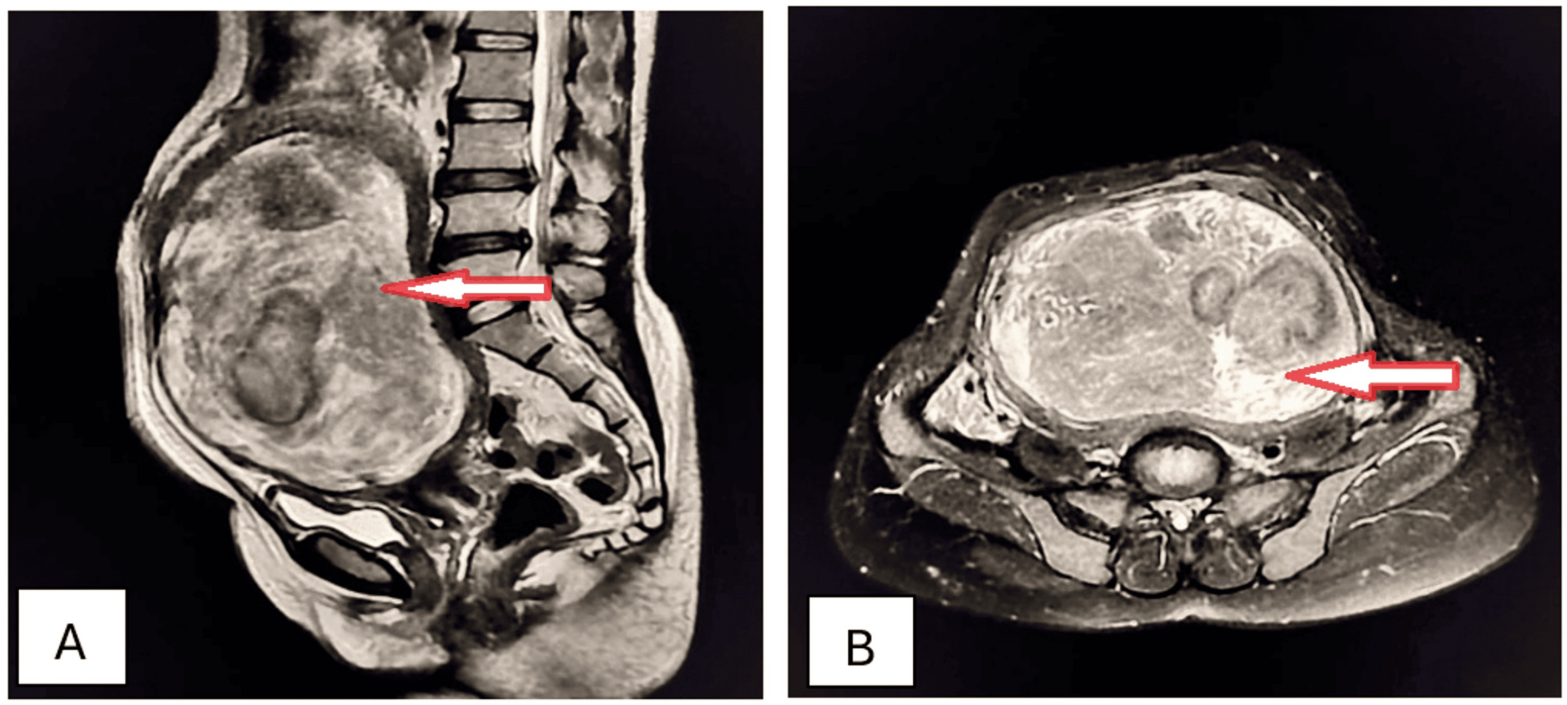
Intramural uterine fibroid with hyaline degeneration (arrows). Sagittal (A) and axial (B) T2W MRI imaging shows a huge, well-defined, inhomogeneous, encapsulated, round-to-oval, hypointense, solid abdominopelvic mass within the myometrium, causing myometrial thinning and exhibiting mixed signal intensity consistent with an intramural fibroid with hyaline degeneration. T2W: T2-weighted
